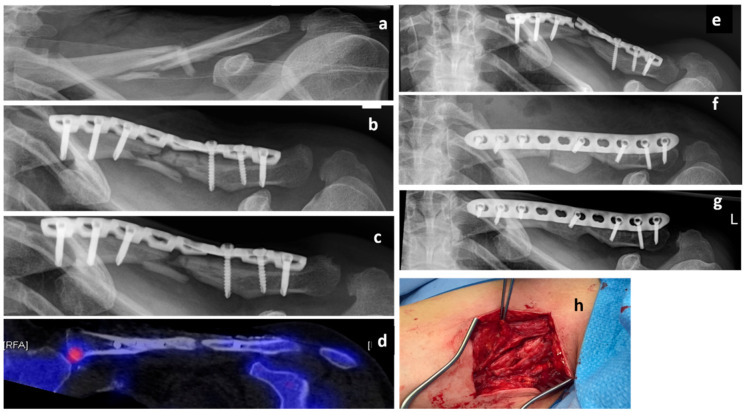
Case 2, x-rays presenting the initial clavicle fracture (a), after open reduction and internal fixation (ORIF) (b), and in the presence of a delayed union 6 months after trauma (c). A single-photon emission computed tomography (SPECT-CT) scan showing a lack of biological activity at the fracture zone 17 months after trauma (d). X-rays of implant failure 18 months after trauma (e), postoperative x-ray after revision osteosynthesis with tricortical iliac bone graft (f), as well as final result 2 years after trauma (g). Intraoperative photographs and showing the atrophic nonunion (h).
